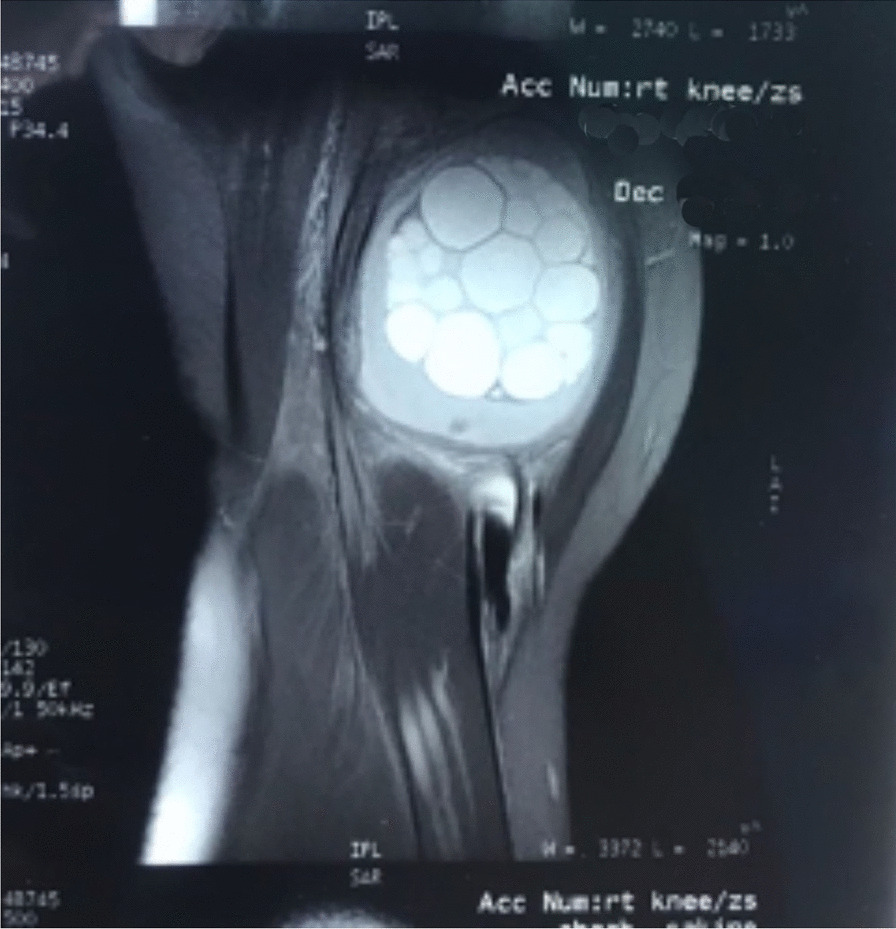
Sagittal T2 weighted knee MRI. The presence of multiple hyper signal daughter cysts and a thick pericyst
